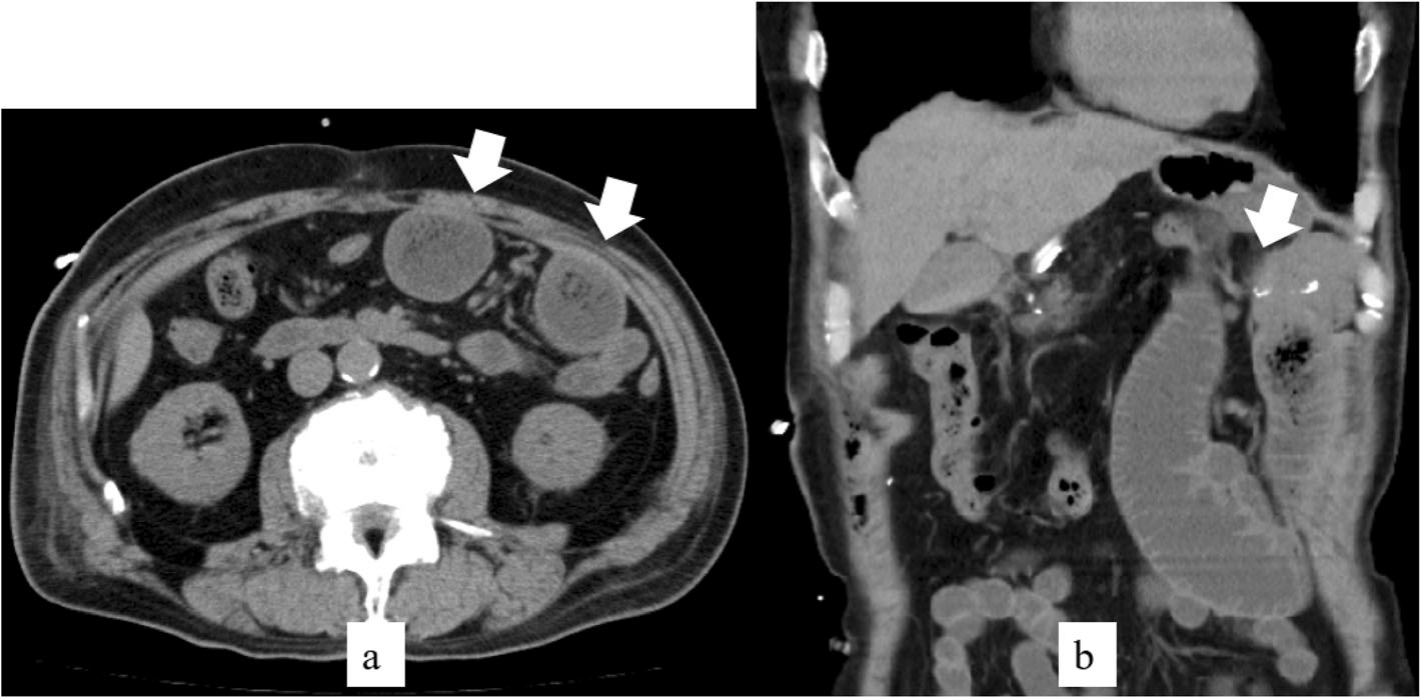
a, b CT scan imaging of jejunojejunostomy obstruction (imaging of case 3). a Dilation of the elevated jejunum (arrows). b Obstruction of the jejunojejunostomy (arrow)
